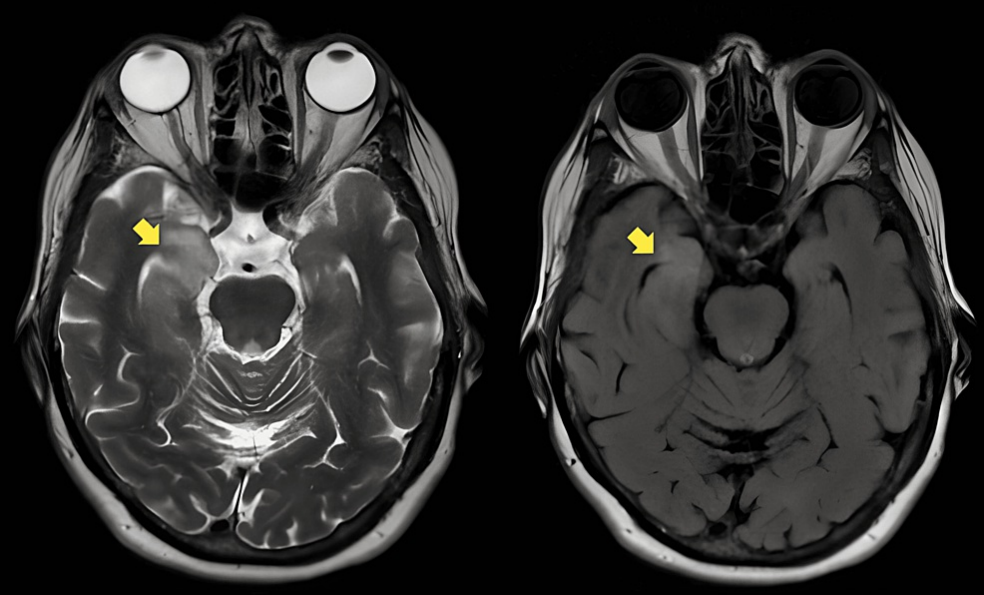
Cranial T2 and T2 FLAIR MR images showing hyperintensity in the right hippocampus and parahippocampus (yellow arrow) FLAIR: fluid-attenuated inversion recovery
